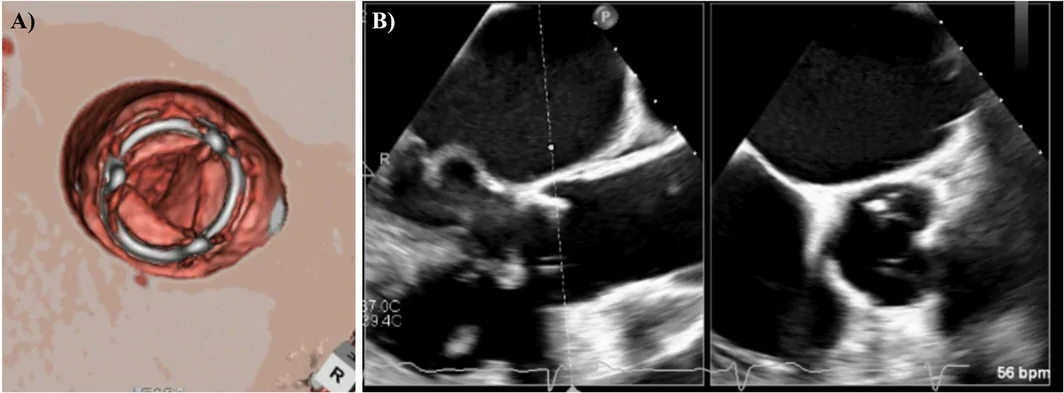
(A) Representative still image from Video S1 showing three‐dimensional reconstructed CT of the bioprosthetic aortic valve. (B) Representative transesophageal echocardiographic image from Video S2 showing the bioprosthetic aortic valve.
